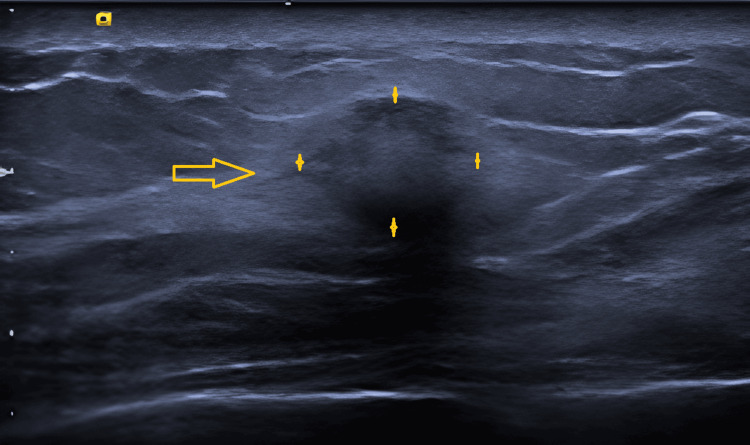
Ultrasound right breast of patient B (arrow indicating lesion as marked between four crosses).
